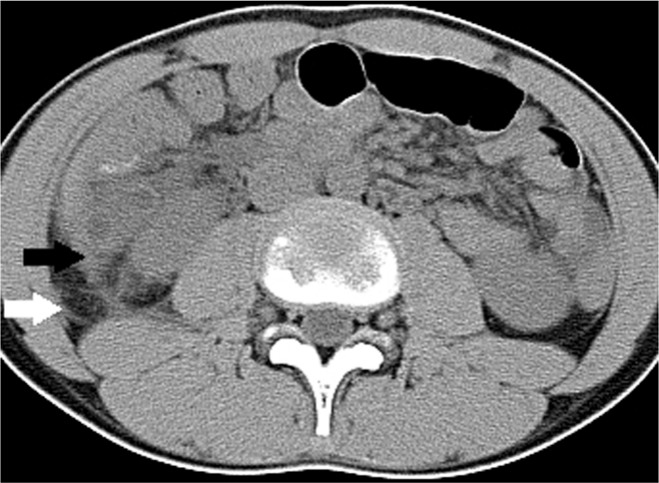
Transverse CT image of 13-year-old boy with complicated appendicitis. The picture shows enlarged appendix with fluid collection in retrorenal plane, subfascial plane (white arrow), lateral conal plane (black arrow), and bridging septa.
